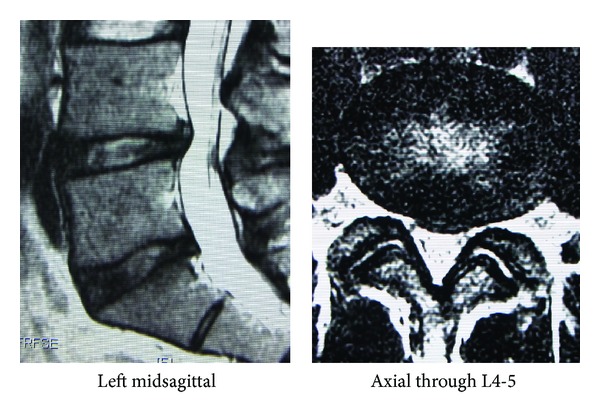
T2-weighted magnetic resonance imaging (MRI) findings on the initial visit. A degenerated disc with a slight protrusion is visible; however, no high signal intensity zone (HIZ) is obvious.
